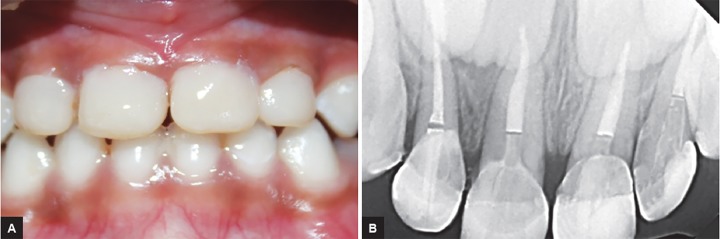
Postoperative intraoral view and radiograph showing restored primary maxillary anterior teeth
